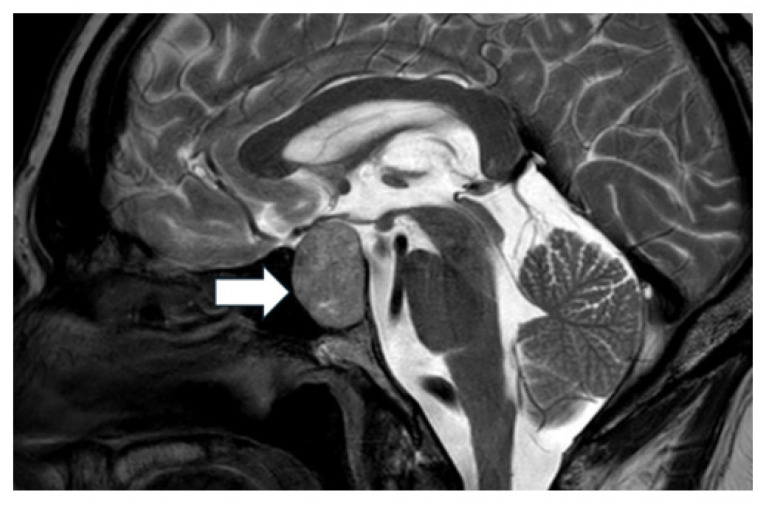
Sagittal T1-weighted contrast-enhanced MRI showing a pituitary macroadenoma (arrow) occupying and expanding the sella turcica with suprasellar extension.
